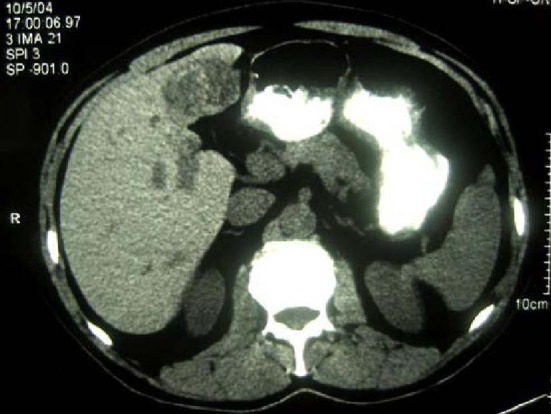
CT scan of liver showing a 4 cm mass in the left lobe with areas of necrosis.
